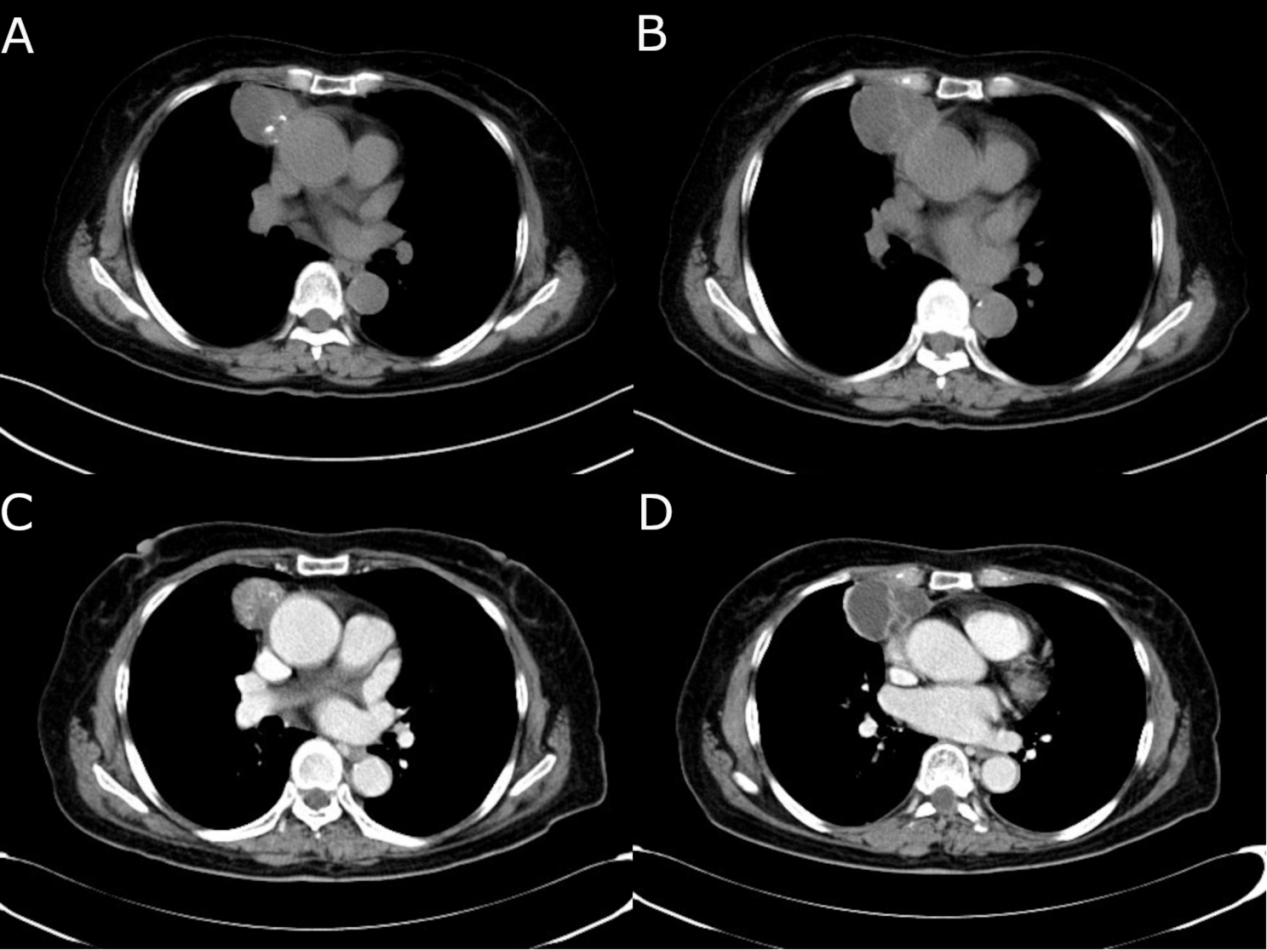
(A and B) A non-contrast chest CT scan revealed a mass in the right anterior mediastinum, measuring approximately 5.0 cm × 4.0 cm, with punctate calcifications noted within. The mass was closely adjacent to the ascending aorta and the right lung lobes. (C and D) Contrast-enhanced chest CT indicated that the mass was cystic and solid, displaying multiple separated cystic spaces, containing soft tissue, calcifications, and cystic components. The mass was well-demarcated from the surrounding tissues and organs
